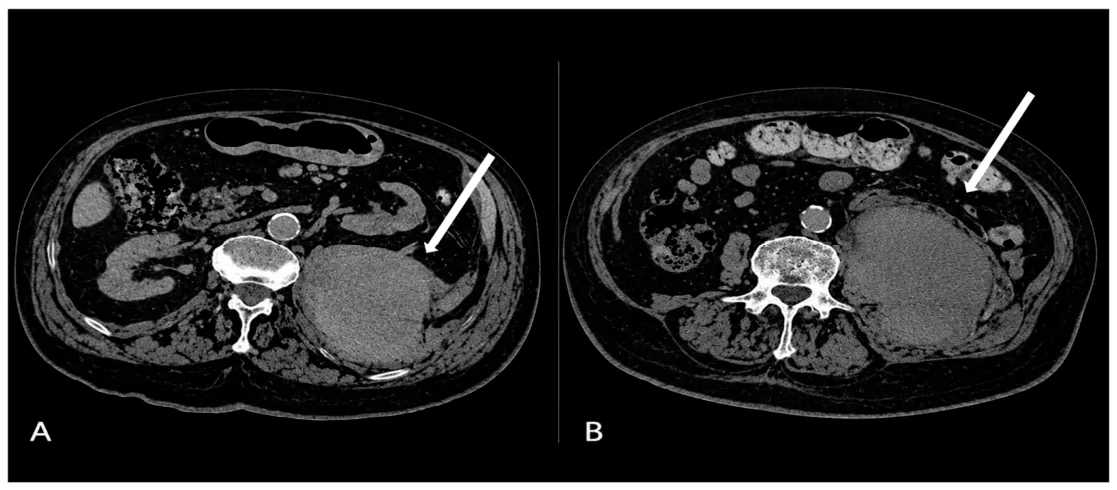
Case 1. A 76-year-old man receiving oral anticoagulant therapy presented with acute left-sided low-back and groin pain, progressive quadriceps weakness, and a reduced left patellar reflex. Axial contrast-enhanced CT images obtained at adjacent levels (A,B) demonstrate a large hematoma (white arrows) expanding the left psoas muscle, in close relationship with the expected course of the lumbar plexus, potentially accounting for the patient’s symptoms. Original Image.
